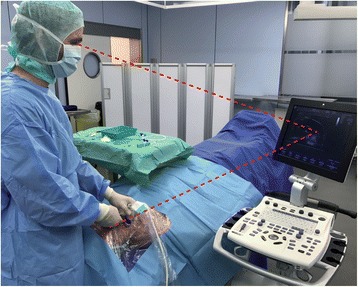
Practical aspects of ultrasound-guided central venous catheter placement in the internal jugular vein using the "single-operator technique”. An aseptic approach including covering the puncture site with a large sterile drape, using sterile barriers (hat, mask, sterile gloves, sterile body gown), and covering the ultrasound probe and cable with a sterile cover is shown. The position of the operator (who holds the ultrasound probe with the nondominant hand while advancing the needle with the dominant hand) allows aligning the insertion site, the needle, and the ultrasound screen in the line of sight during needle insertion (red lines) (Color figure online)
